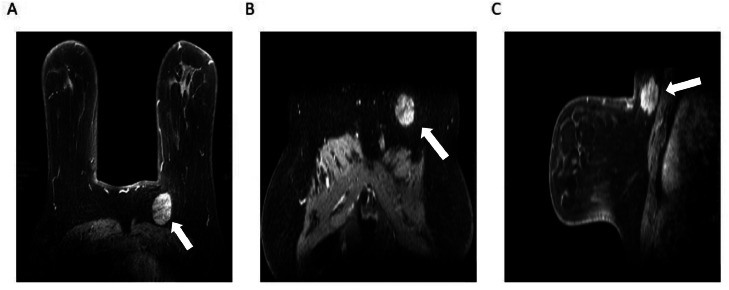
Contrast-enhanced fat-suppressed MRI: Left breast mass shows heterogeneous enhancement (Panel A: axial view, Panel B: coronal view, Panel C: lateral view, mass location indicated by white arrow).
